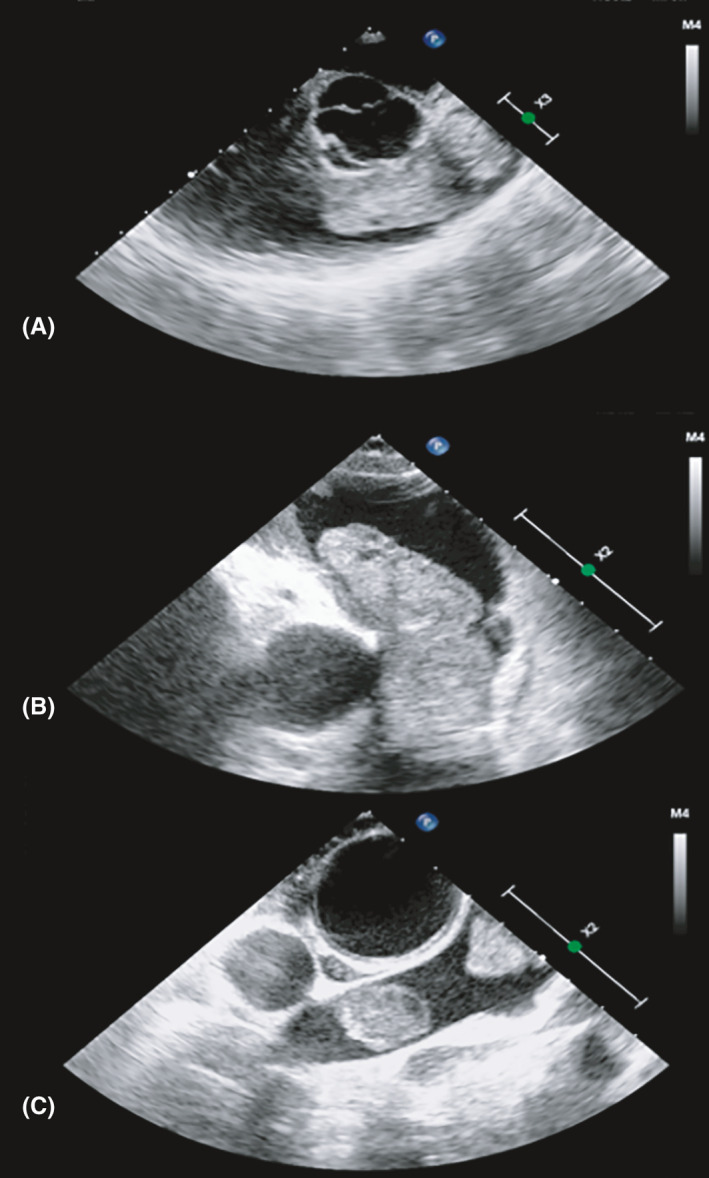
Intraoperative transoesophageal echocardiography. A and B, coarse and inhomogeneous mass of the right ventricular outflow tract. C, mass involving the right branch of the pulmonary artery
